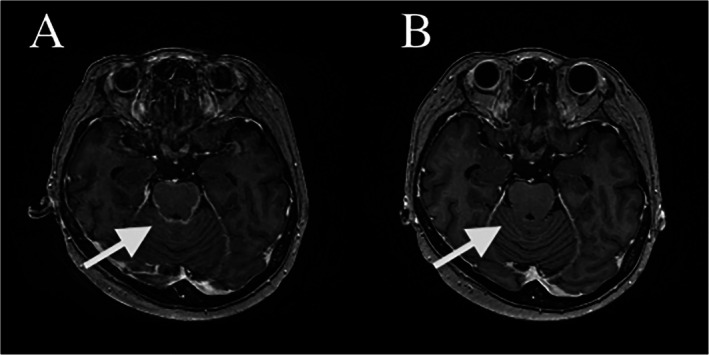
(A) Baseline contrast‐enhanced MRI showing diffuse leptomeningeal carcinomatosis (arrow). (B) Fourteen days after palliative osimertinib rechallenge, leptomeningeal enhancement markedly improved (arrow).
